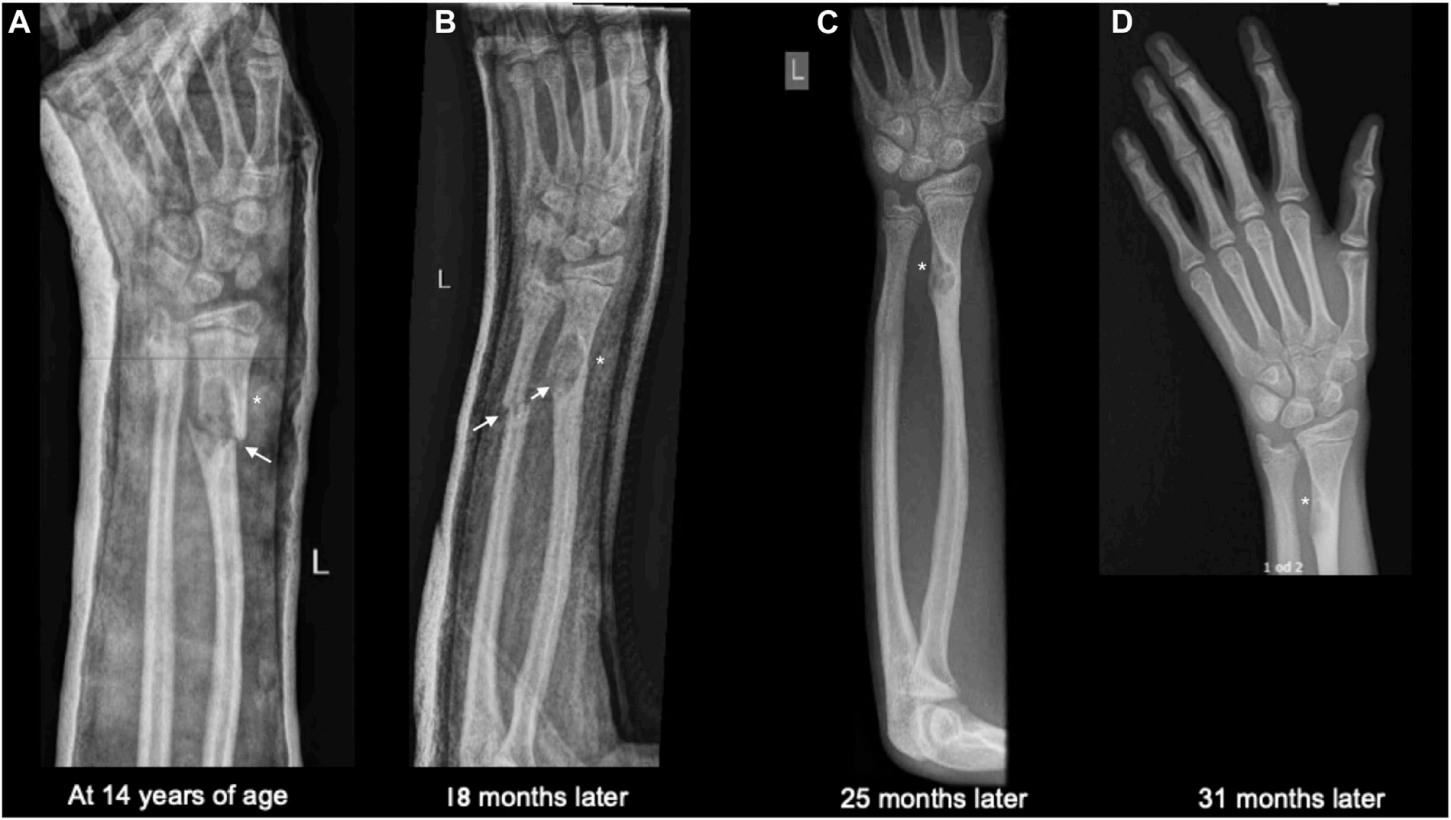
(A) Trauma anteroposterior radiograph of the right upper extremity showing a fracture through lesion in the distal radius and a fracture of ulna. (B) Trauma anteroposterior radiograph showing second fracture in the same area 18 months later. (C,D) Anteroposterior radiograph of the upper extremity 25 months (C) and 31 months (D) after the first injury showing united fracture. Fractures are indicated by arrows and non-ossifying fibromas by asterisks.
